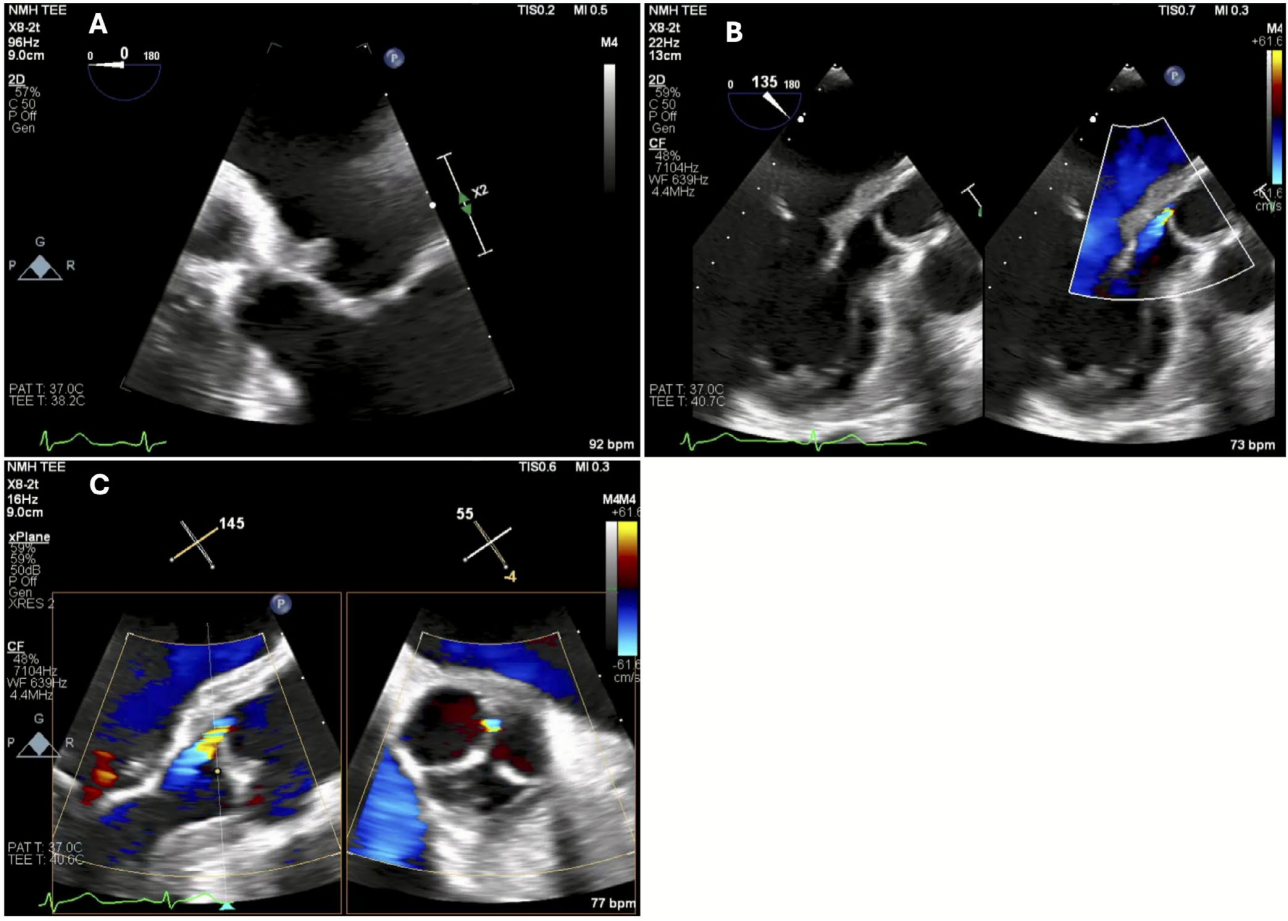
Two‐dimensional (B‐mode) transesophageal echocardiographic (TEE) still‐frames demonstrating: (A) a mobile echodensity (1.1 × 0.7 cm) attached to the anterior mitral annulus at the aortomitral curtain, consistent with vegetation; (B) heterogenous tissue extending into the aortic root, compatible with periannular abscess formation; and (C) mild aortic regurgitation originating between the left and non‐coronary cusps, with possible cusp perforation and a small filamentous structure on the aortic side of the valve suggestive of Lambl's excrescence or vegetation.
